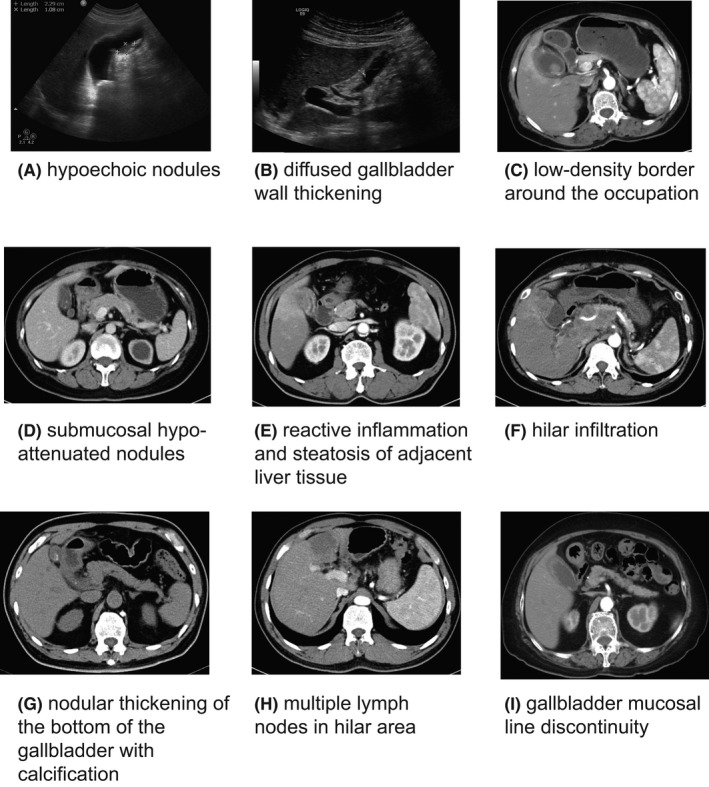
Xanthogranulomatous cholecystitis (XGC) and gallbladder carcinoma (GBC)’s characteristics in ultrasonography and computed tomography (CT) scan. Each of the disclosures in the figure has a corresponding figure. (A) hypoechoic nodules observed in XGC patients under ultrasound. (B) diffused gallbladder wall thickening observed in XGC patients under ultrasound. (C) low density border around the occupation. (D) submucosal hypo‐attenuated nodules. (E) reactive inflammation and steatosis of adjacent liver tissue. (F) hilar infiltration. (G) nodular thickening of the bottom of the gallbladder with calcification. (H) multiple lymph nodes in hilar area. (I) gallbladder mucosal line discontinuity
